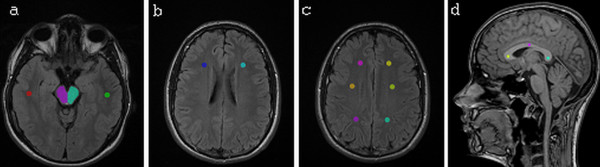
Regions of interest drawn on the each level of interest. Figure 1a: an axial FLAIR (T2w FLAIR) image in level 1. Figure 1b: an axial FLAIR (T2w FLAIR) image in level 2. Figure 1c: an axial FLAIR (T2w FLAIR) image in level 3. Figure 1d: sagittal T1w 3D magnetization prepared gradient echo (T1w MPR) in level 4. Regions of interest (ROIs) are drawn in the images.
